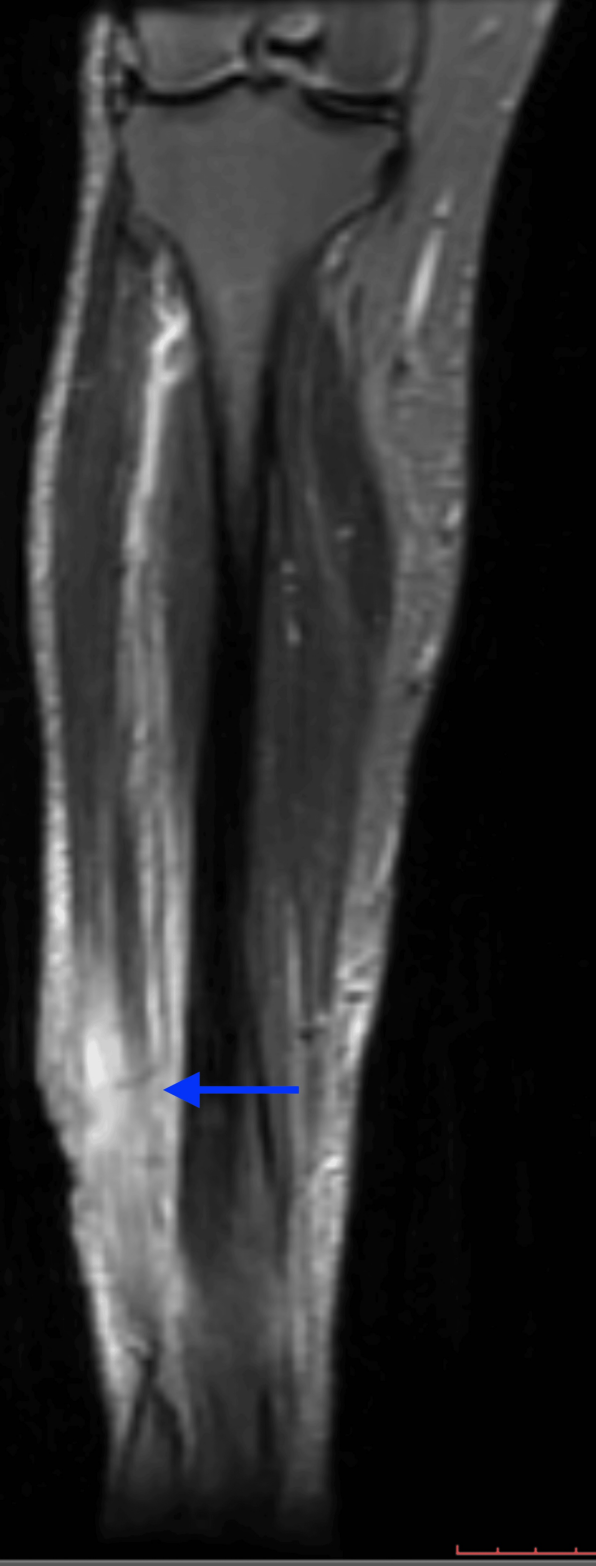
Coronal view of the T2-weighted MRI of the right lower extremity demonstrating area of inflammation and infection (blue arrow) MRI: magnetic resonance imaging
